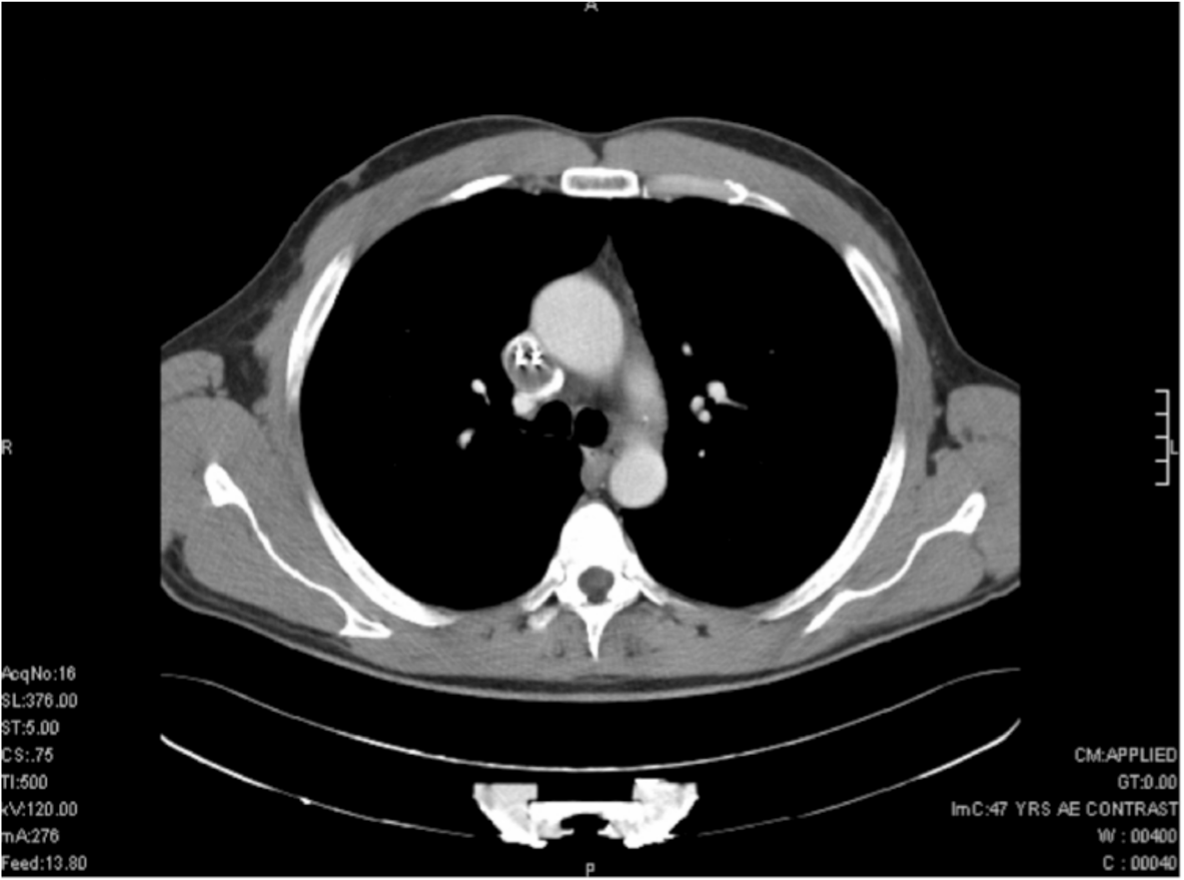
Transverse plane image of contrast-enhanced CT scan demonstrating two pacemaker leads within the SVC.
